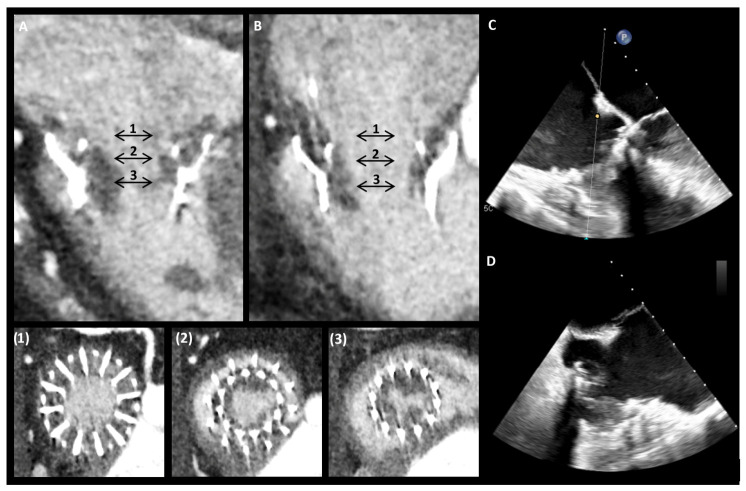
Clinically relevant thrombosis of a transcatheter tricuspid prosthesis. Computed tomography showed diffuse thrombotic involvement of the prosthesis ((A): long-axis, 4-chamber view; (B): long-axis, 3-chamber view; short-axis sections are reported in Figures (1)–(3)). Thrombus was also detectable by transesophageal echocardiography ((C): mid-esophageal view, 0°; (D): mid-esophageal view, 155°).
